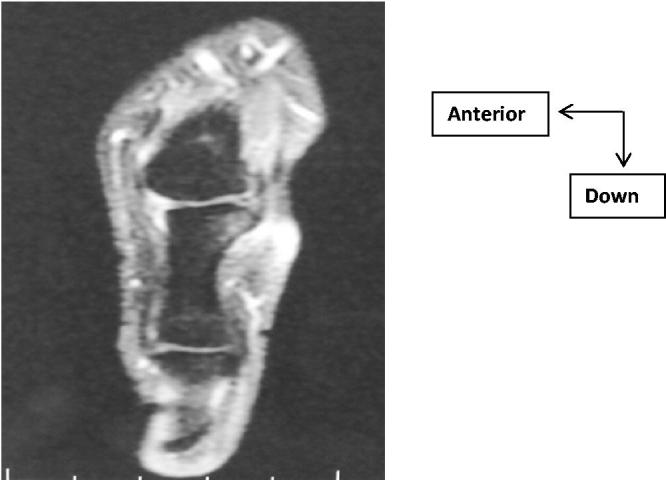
MRI: (sagittal section fat sat T2) Thickened aspect of LCL of MCPP which is in Hypo-signal T1 Hypo-signal T2.
